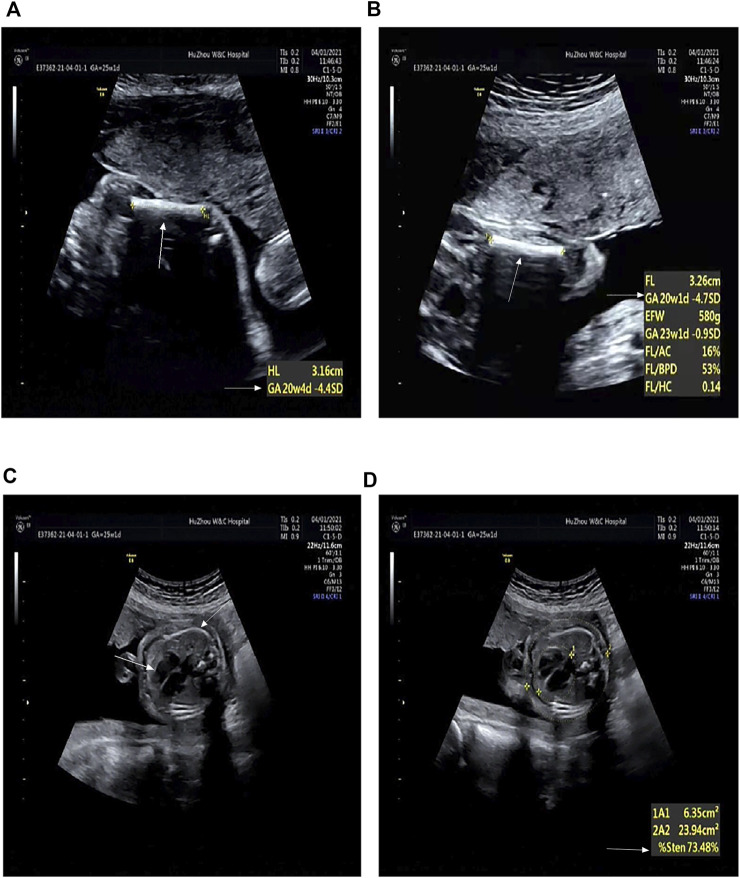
Radiographic features of the first fetus (at 25+1 weeks’ gestation). (A) Humerus is smaller by 4.4 SD (arrows). (B) Femur is smaller by 4.7 SD (arrows). (C) Thoracic area is significantly reduced (arrows). (D) Enlarged cardiothoracic ratio (arrows).
